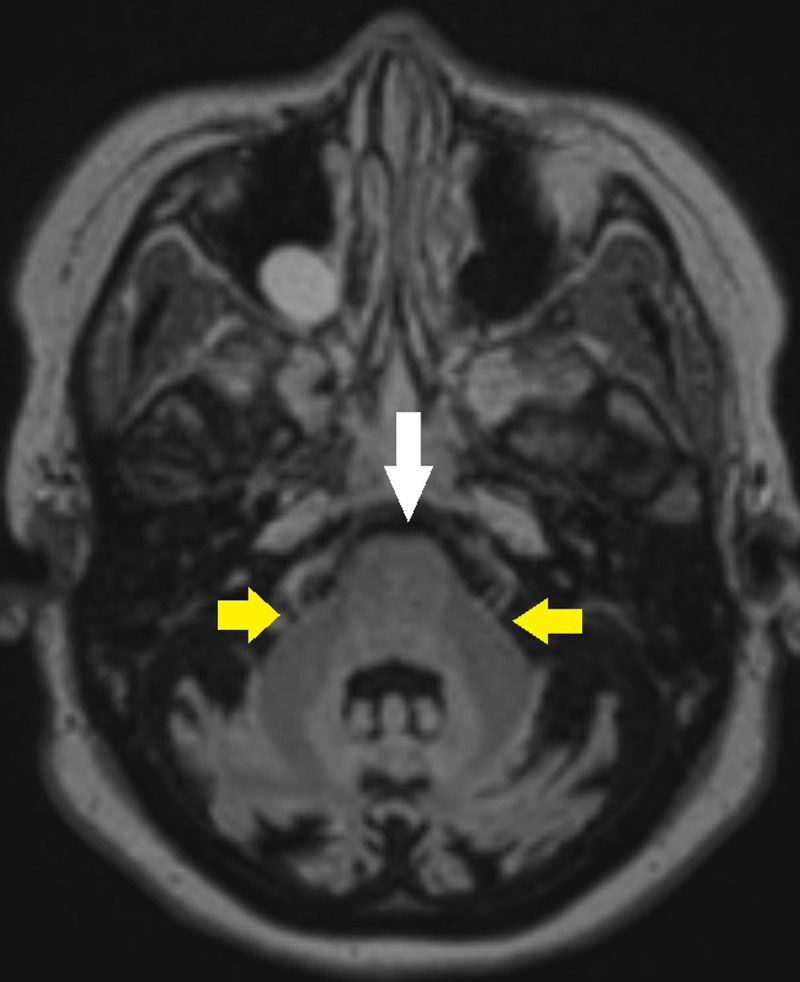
MRI T2 FLAIR axial image showing atrophy of the pons (white arrow) and relatively preserved middle cerebellar peduncles (yellow arrows).
